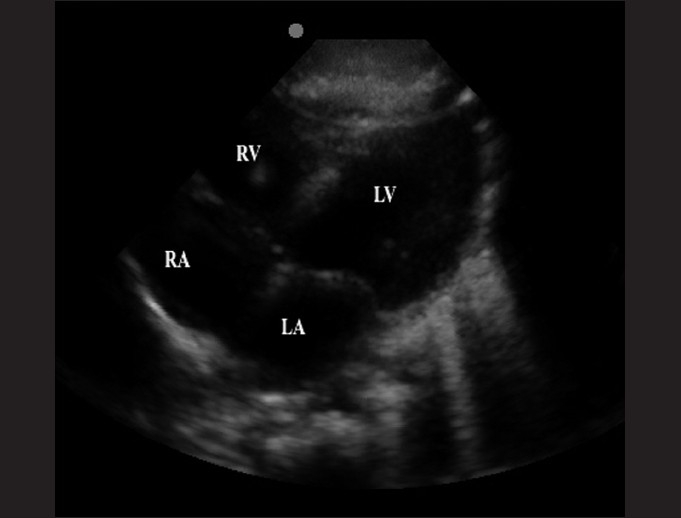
The apical 4-chamber view (RV: right ventricle; LV: left ventricle; RA: right atrium; LA: left atrium)
